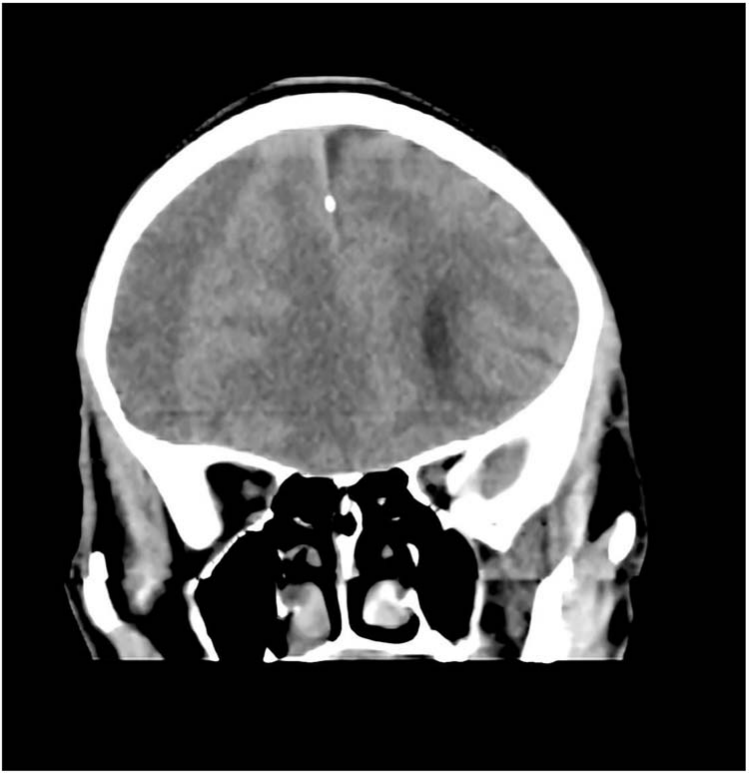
Coronal CT with right CSDH.
